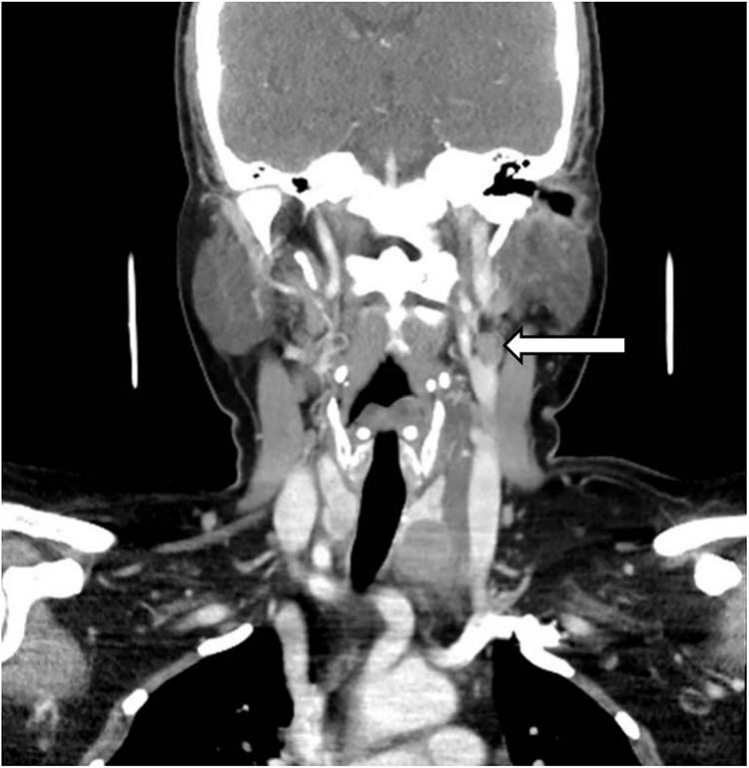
Coronal section of CT neck, showing a anteromedial inflammation around the carotid bifurcation (white arrow).
